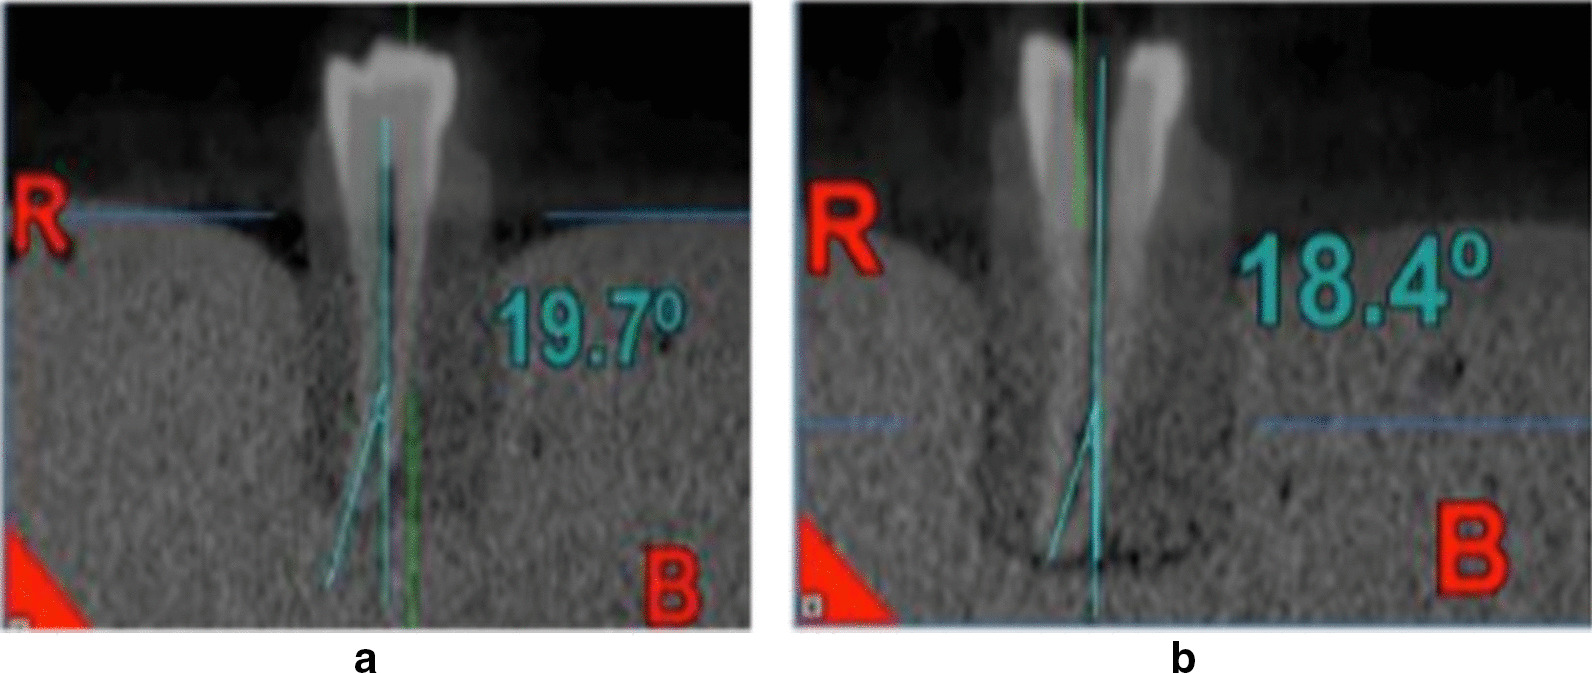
Representative CBCT images demonstrating a post-instrumentation canal curvature angle measurement
